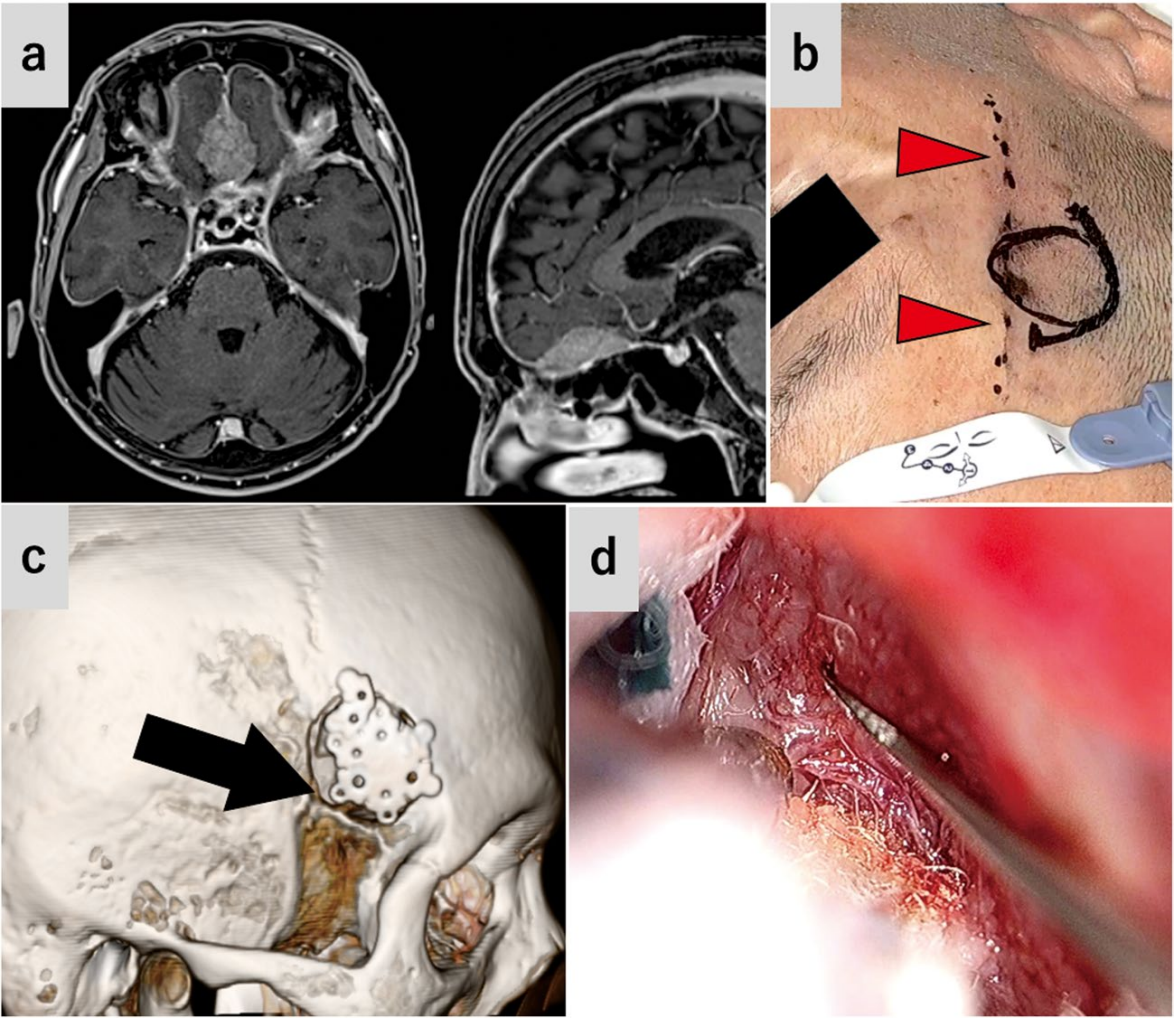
Case 2: Olfactory groove meningioma (a) Preoperative gadolinium-enhanced T1-weighted MRI. b Preoperative image. The solid line shows the designed skin incision and craniotomy size. Red arrowheads, the temporal branch of facial nerve marked by a dotted line. c Postoperative 3D computed tomography shows craniotomy. Black arrow, ICP. d The posterior part of the tumor attaching the planum sphenoidale was exoscopically removed
